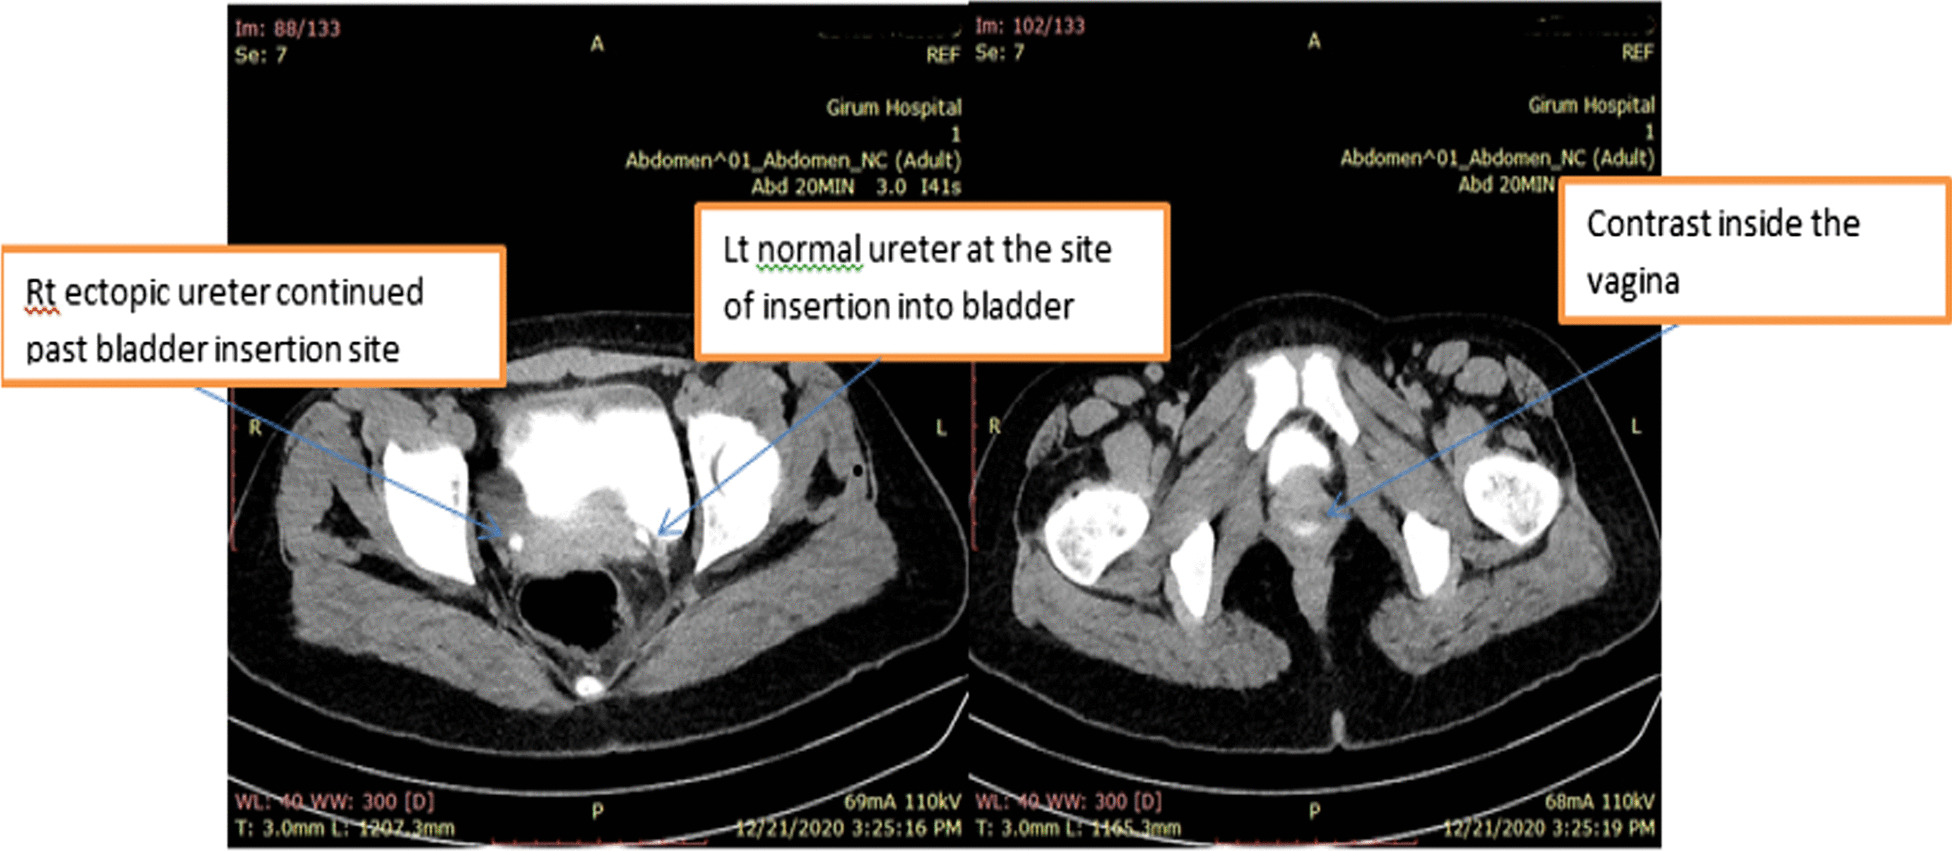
CT scan with contrast image shows right ectopic ureter and left normal ureter at site of bladder insertion (left side) and contrast tracking into the vagina (right side)
